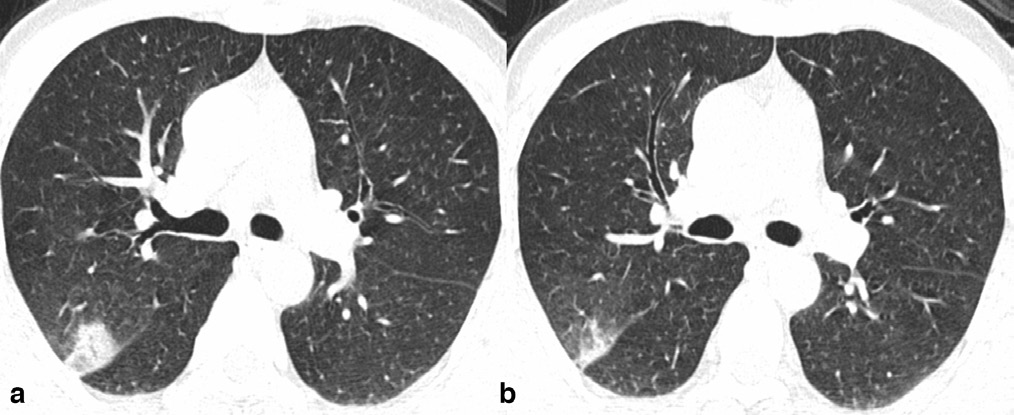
Axialchest CT images in lung window settings of a 46-year-old COVID-19 asymptomaticpatient showing a patch of consolidation in posterior segment of right upperlobe at admission (A). Follow-up CT performed 10 days latter reveal reductionin the size of opacity (B).
